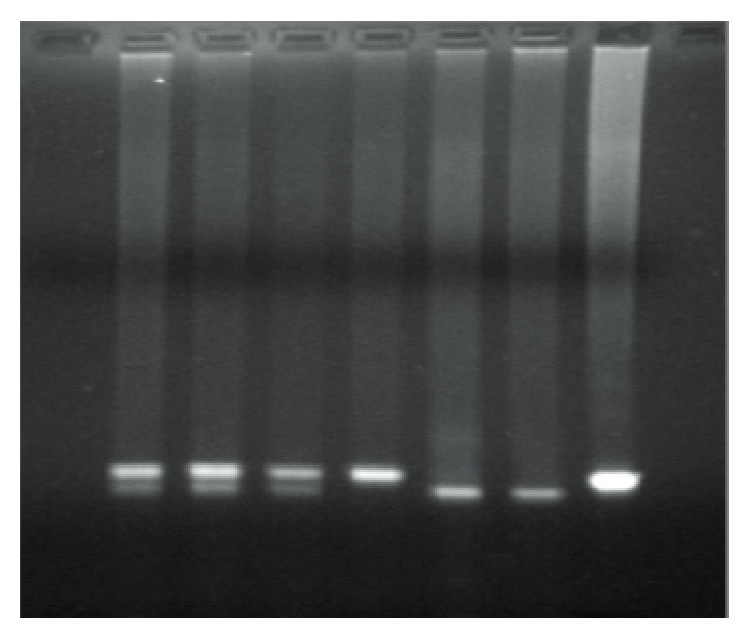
Electrophoresis of digestion product. Genotypes are visualized as follows: on lines 1, 2, and 3 CT, on line 4 TT, on lines 5, 6 CC, and on line 7 DNA sample control.
